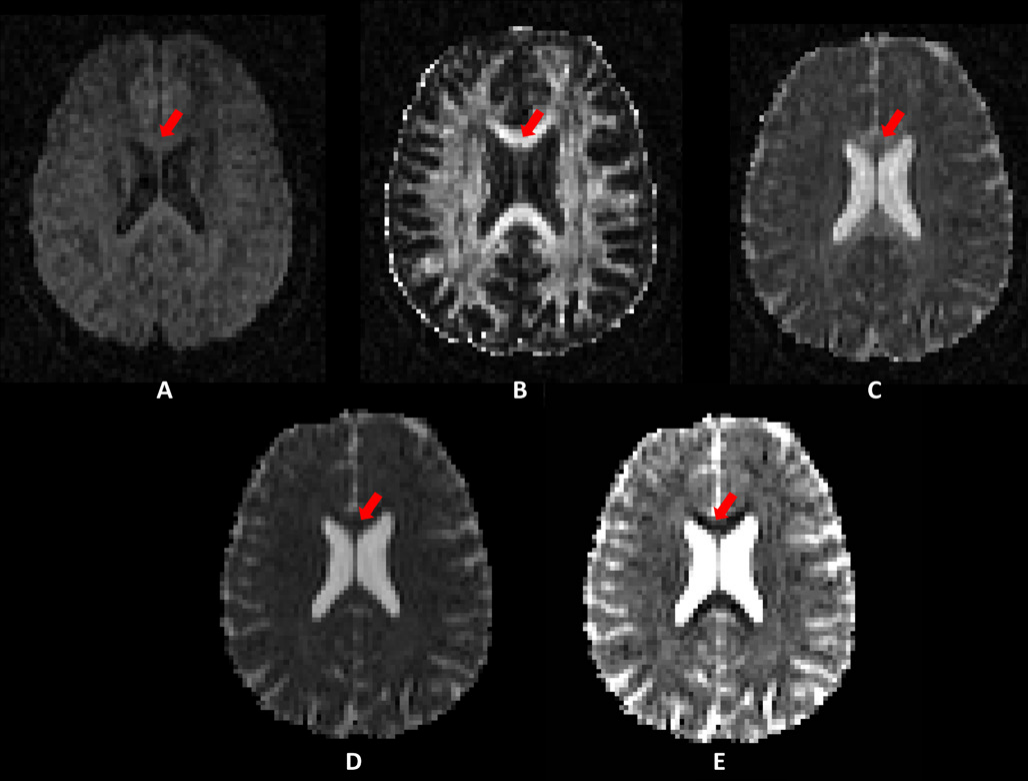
(A)Diffusion weighted imaging raw image; (B) fractional anisotropy map; (C) axial diffusivity map; (D) mean diffusivity map; and (E) radial diffusivity map from one participant. Note the differences in areas with high white matter coherence such as in the corpus callosum (red arrows)DWI, Diffusion-weighted imaging; DTI, Diffusion Tensor Imaging.
